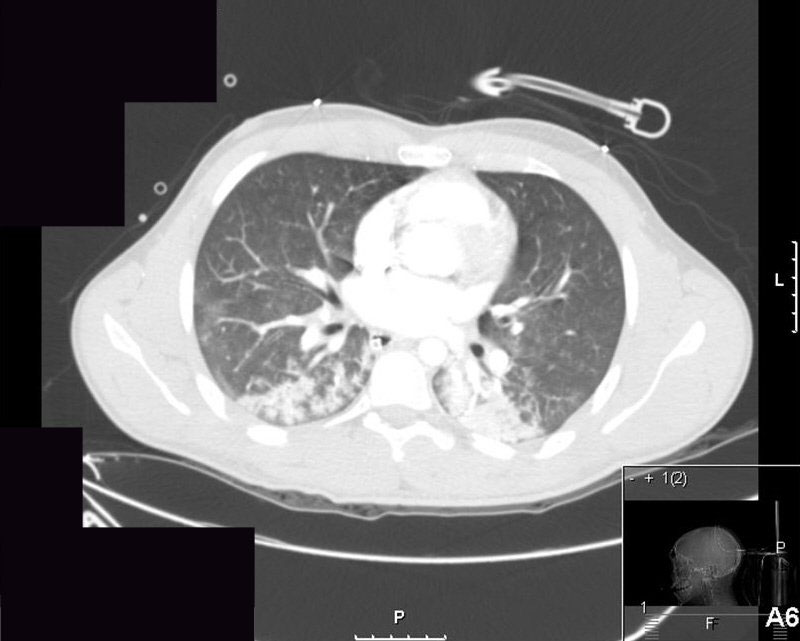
CT scan of the chest showing bilateral, bibasilar infiltrates.
